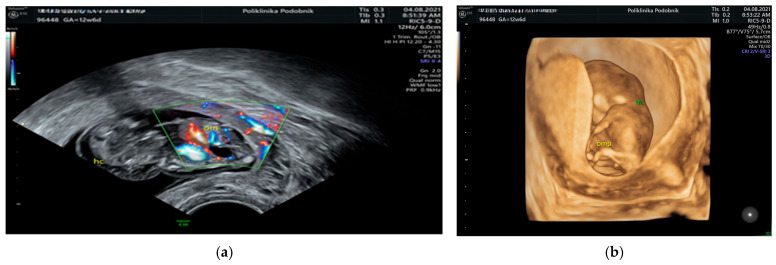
Ultrasonographic image of a septated fetal cystic hygroma with associated omphalocele using color Doppler imaging (a) and corresponding 3D transvaginal ultrasound. (b) The fetus was at 12.6 gestational weeks and karyotyping revealed trisomy 13.
